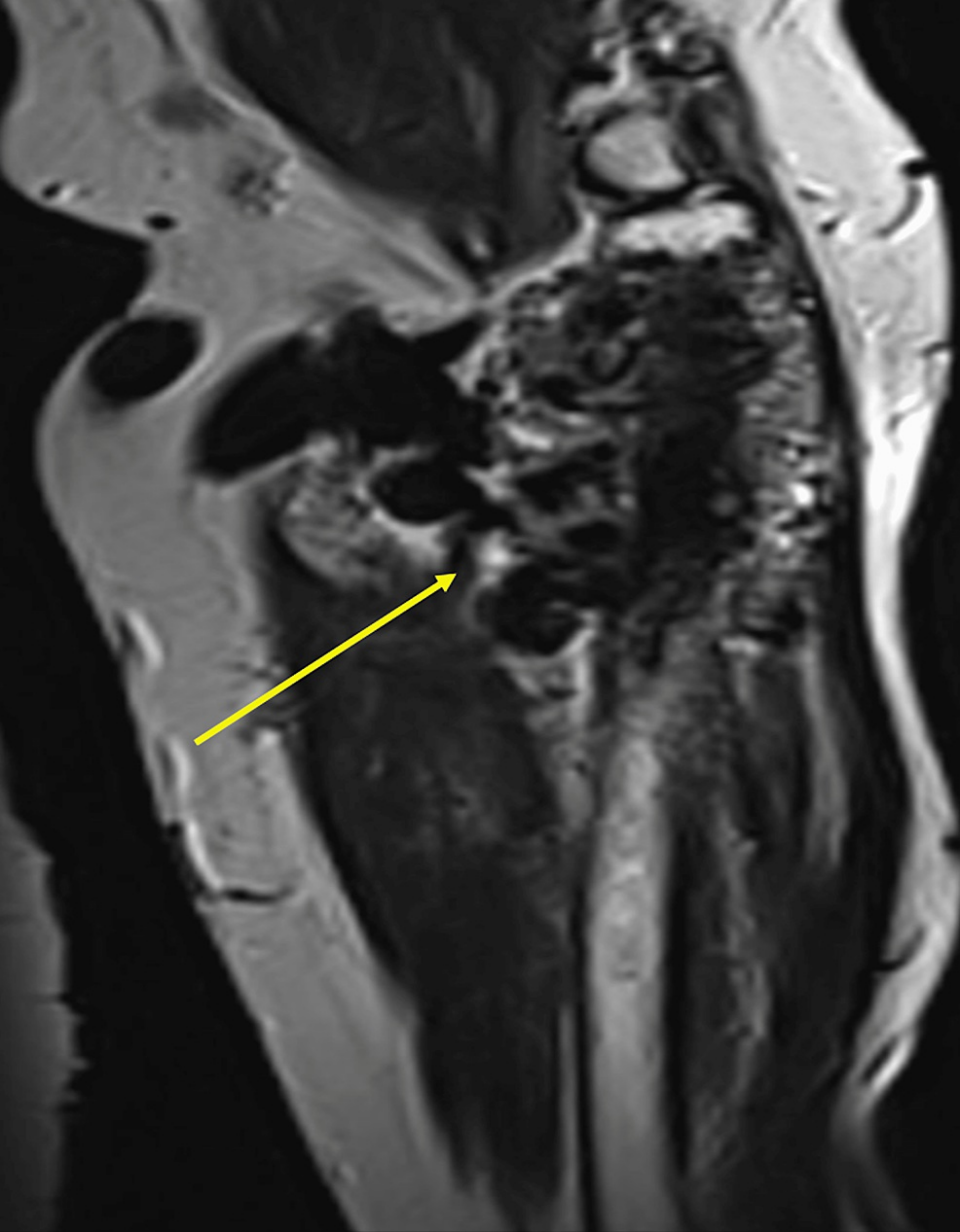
Magnetic resonance imaging (MRI) showing infiltration of the proximal radius bone
